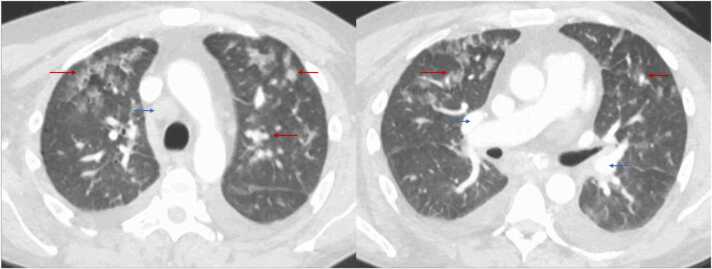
Computed tomography of the Chest with contrast revealed multifocal innumerable upper lobe predominant ground glass and nodular opacity (Red arrows) in bronchovascular distribution with mild pulmonary edema and reactive enlarged mediastinal and hilar lymph nodes (Blue arrows).
